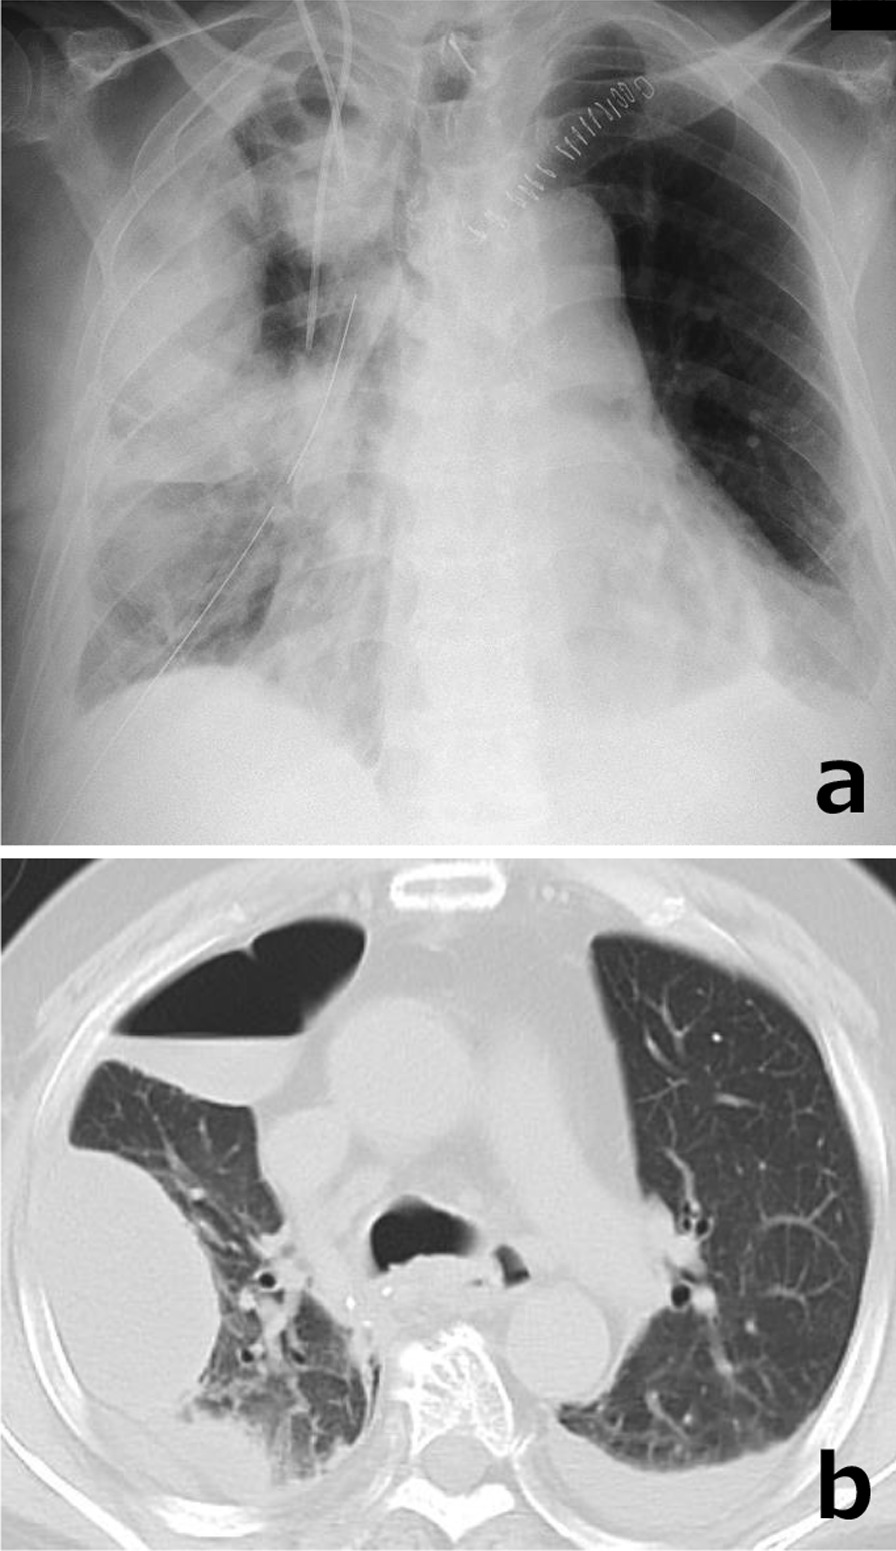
Chest X-ray and CT images on POD 7. a Lobar pneumonia of the right lung and pyothorax of the right middle and upper lobes. b Multiple pyothoraces, pneumonia and pneumothorax of the right lung and bilateral pleural effusion
